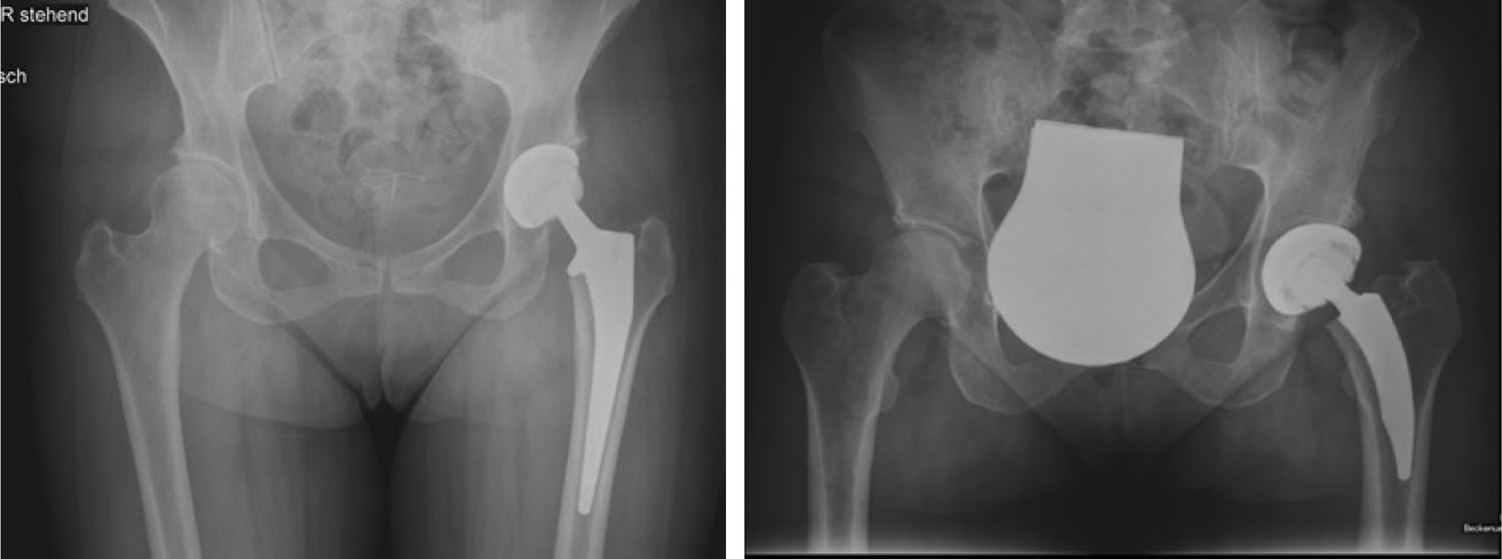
a Anteroposterior radiograph of the pelvis after THA with a standard stem (Corail^®^, DePuy, Warsaw, IN). b Anteroposterior radiograph of the pelvis after THA with a short stem (Nanos^®^, Smith & Nephew, Marl, Germany), *THA* total hip arthroplasty
